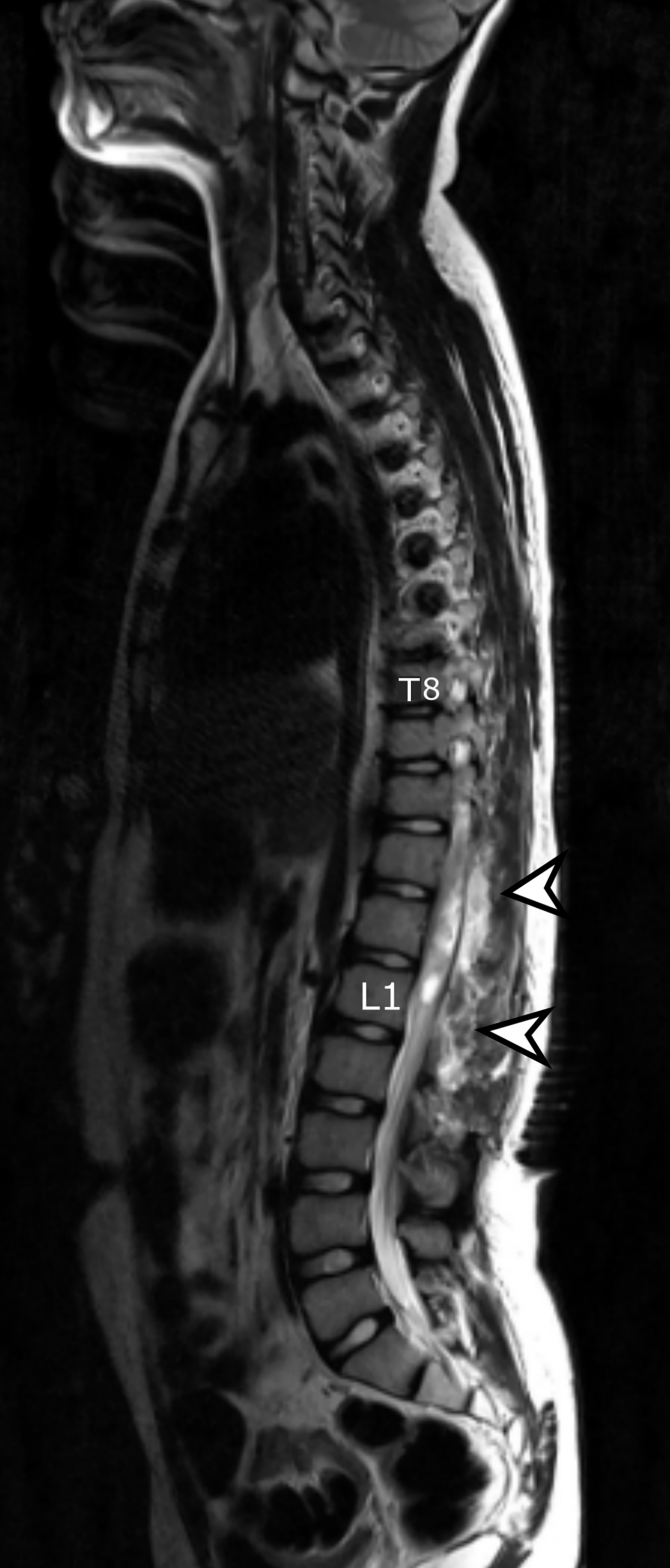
Immediate post-operative MRI spine, sagittal view. T2 weighted image showing hyperintense signals identified extending proximally in the thoracic spinal cord representing cord edema due to post-surgical changes (arrows). MRI: Magnetic resonance imaging.
